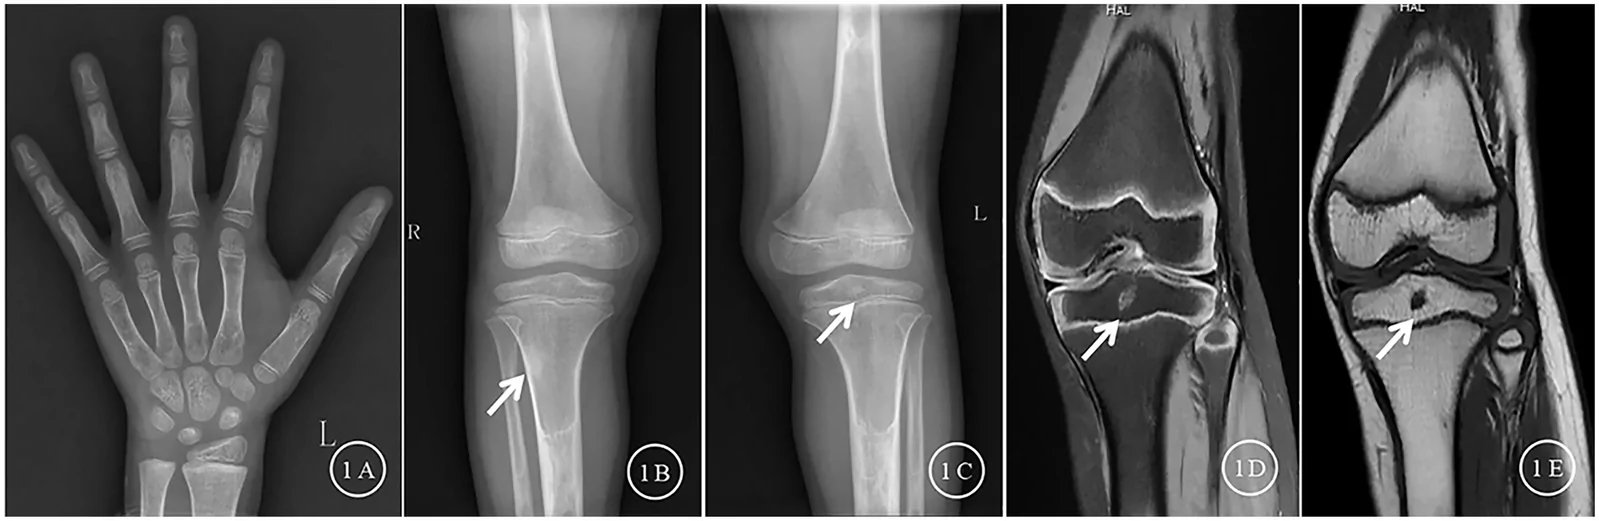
Pediatric ECD with epiphyseal involvement. (A) Bone age X-ray: chronological age 11 years 5 months, R-series bone age 8 years 3 months, and C-series bone age 8 years 7 months. (B,C) X-ray of both knees: patchy high-density shadows in the left tibial epiphysis and lateral edge of the right tibial metaphysis (white arrows). (D,E) MRI of the left knee showing: patchy hypointensity on T1WI and hyperintensity on T2WI in the left tibial epiphysis with clear margins (white arrows).
